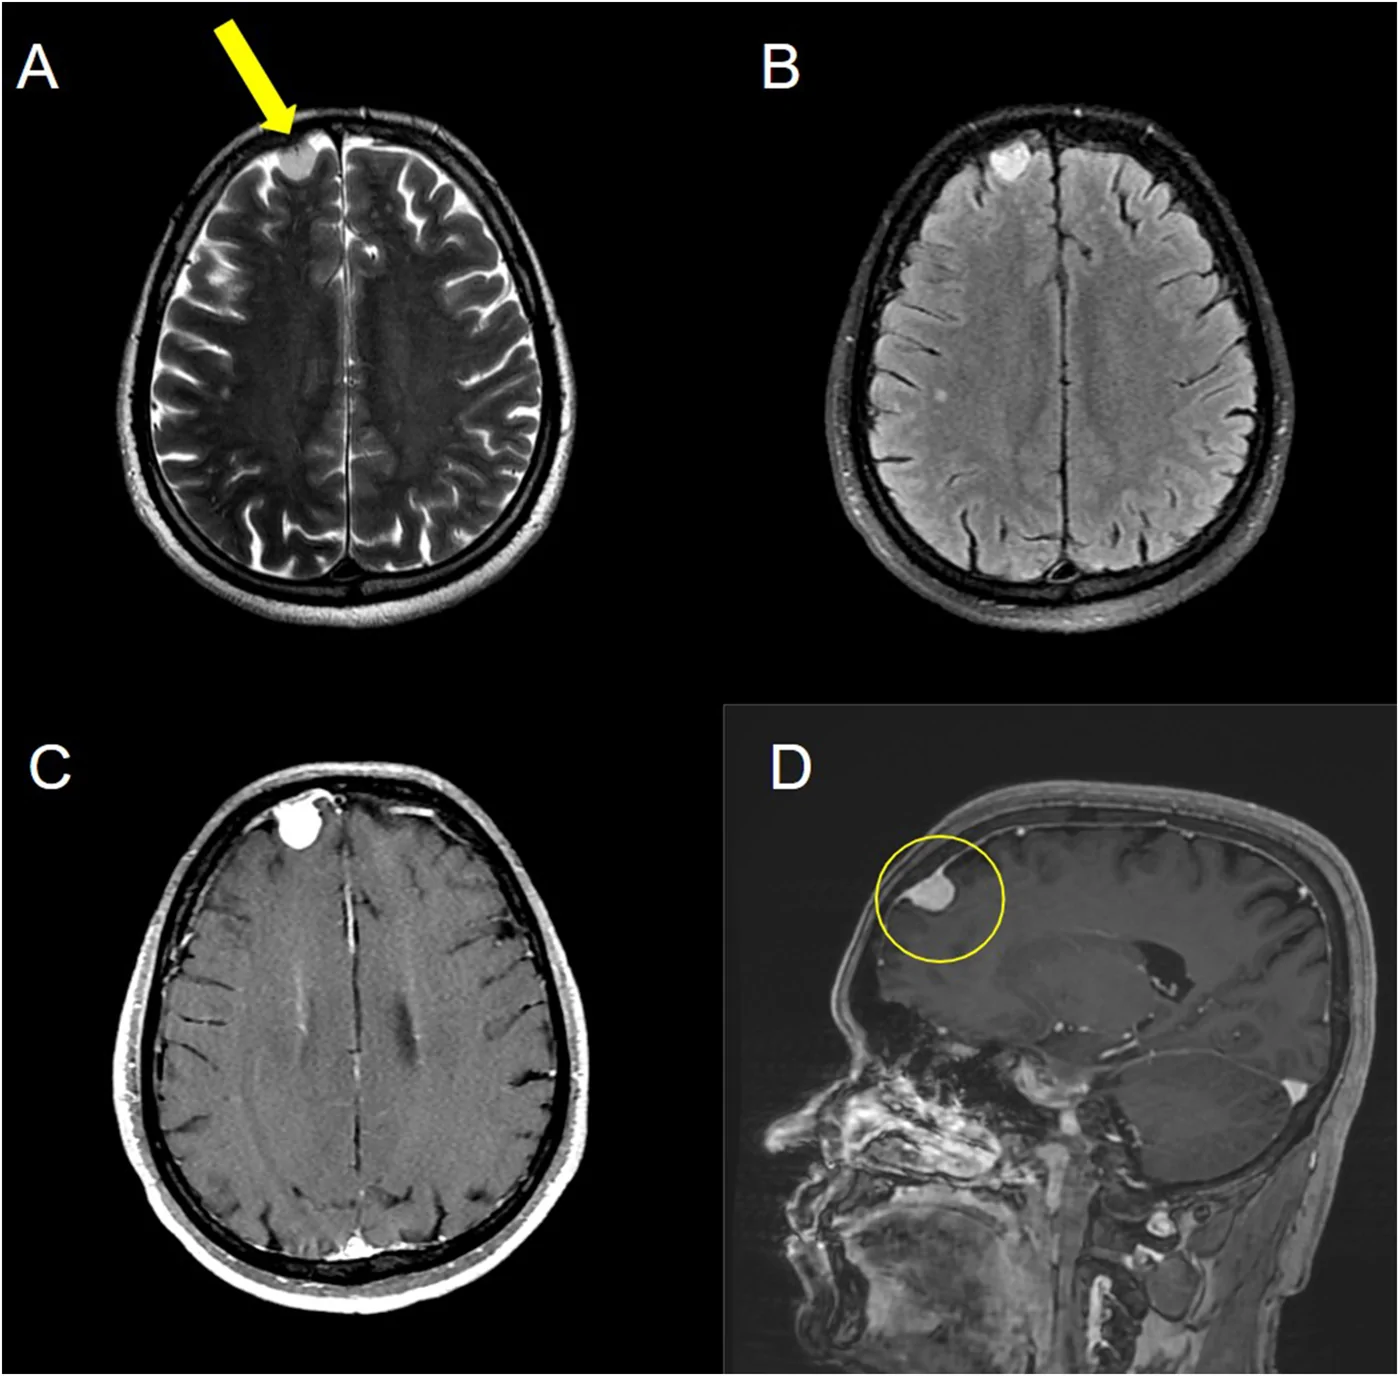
Meningioma. (A) Axial T2-weighted image shows a well-circumscribed, solid extra-axial hyperintense lesion in the right anterior frontal region (yellow arrow) without significant surrounding vasogenic edema or mass effect. (B) Axial FLAIR image demonstrates the lesion to be hyperintense relative to adjacent brain parenchyma. (C) Axial T1-weighted post-contrast image reveals homogeneous solid enhancement with a characteristic dural tail extending along the adjacent dura mater. (D) Sagittal T1-weighted post-contrast image confirms avid homogeneous enhancement and the presence of a dural tail (yellow circle), consistent with a dural-based extra-axial mass such as a meningioma.
